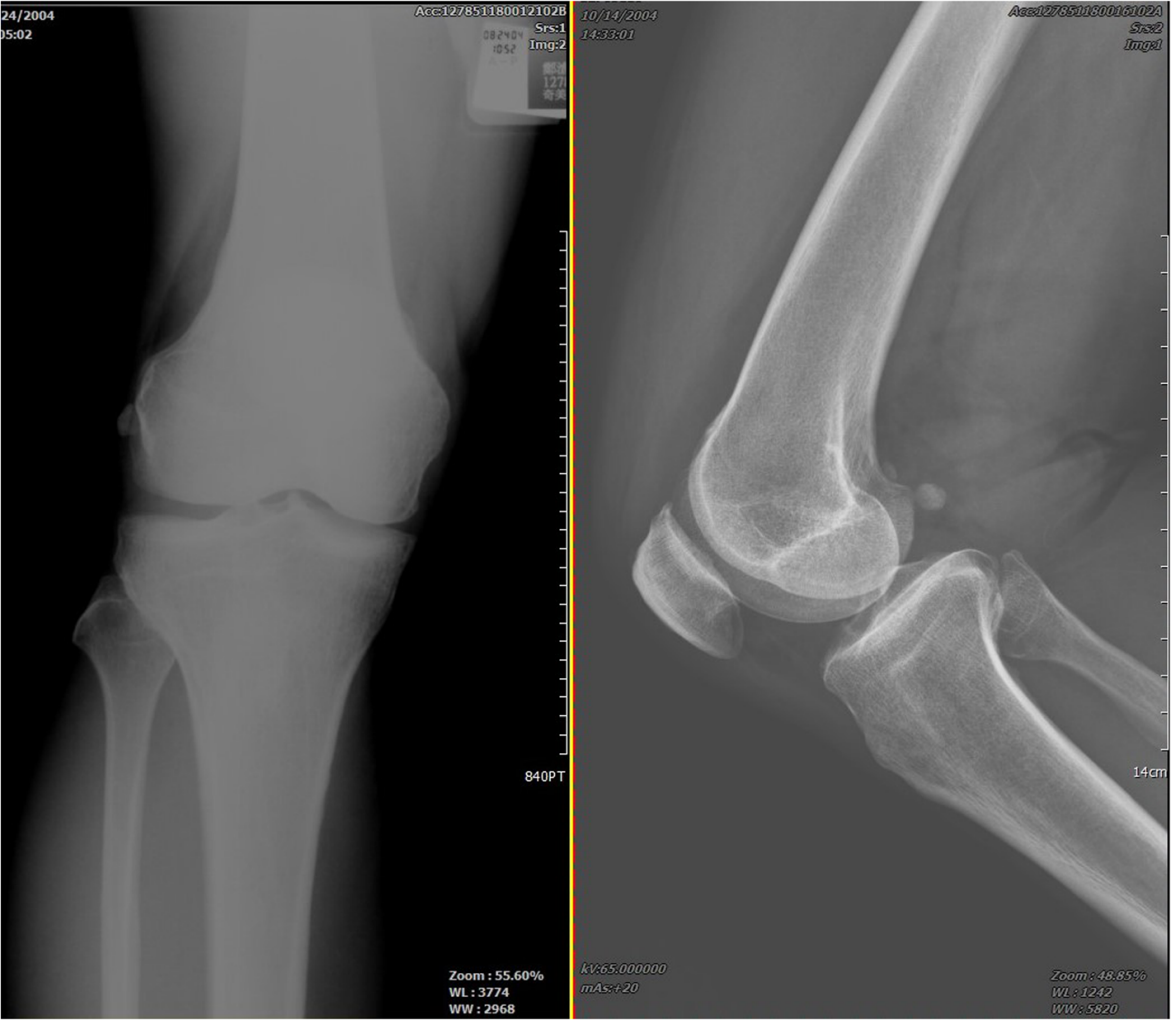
The right knee X-ray showed mild osteoarthritis with a significant fabella bone at the posterolateral side of knee
